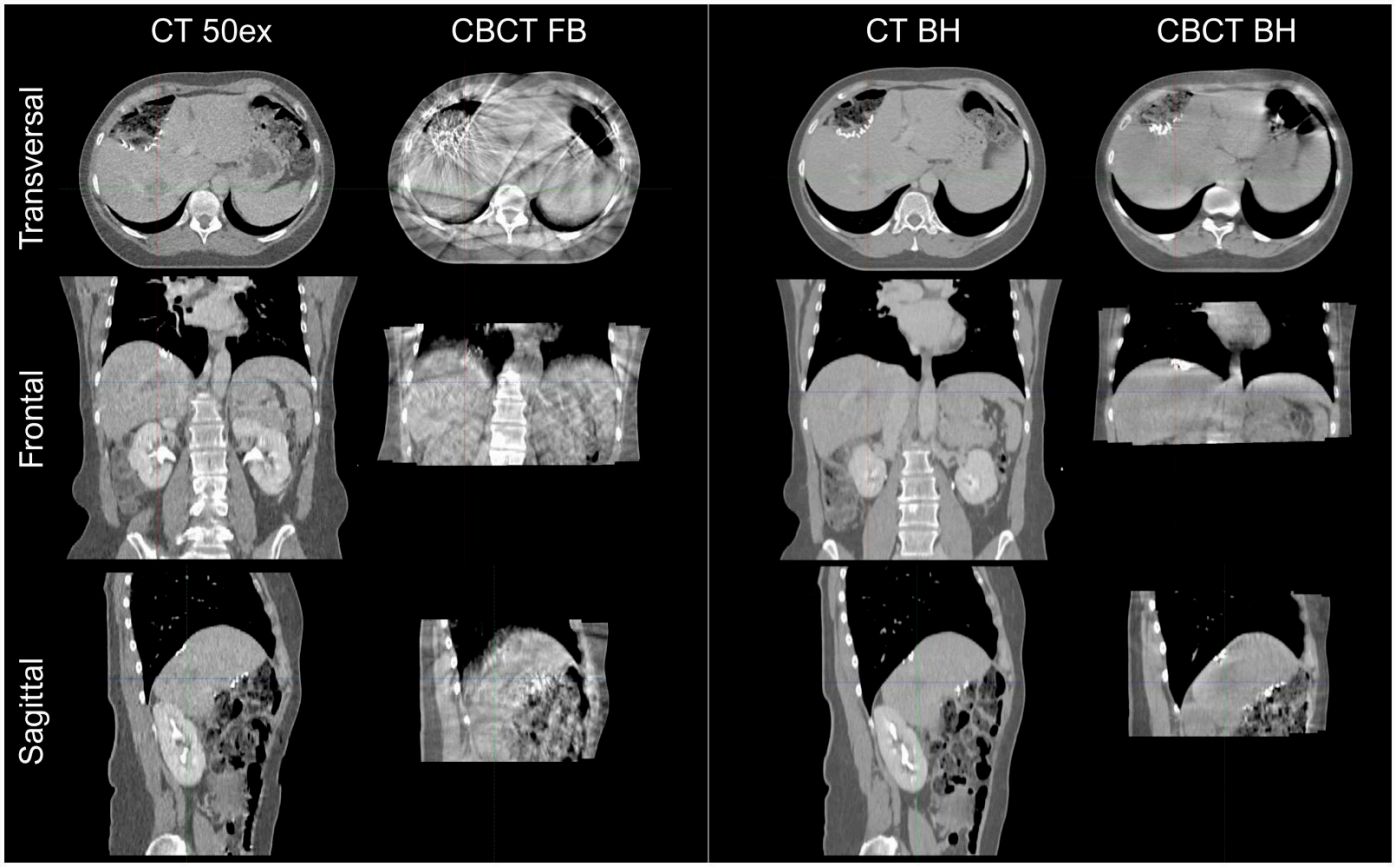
Example of a free-breathing (FB) planning CT scan, which is the 50% exhale phase of a 4DCT scan, a cone-beam CT (CBCT) scan acquired in free-breathing, a breath-hold (BH) CT scan, and a breath-hold CBCT scan. These scans were acquired of a pilot patient, in which both CBCT scans were acquired during the same treatment fraction. In the free-breathing CBCT scan, blurring and artifacts are observed due to breathing motion, which disappeared for the breath-hold CBCT scan.
